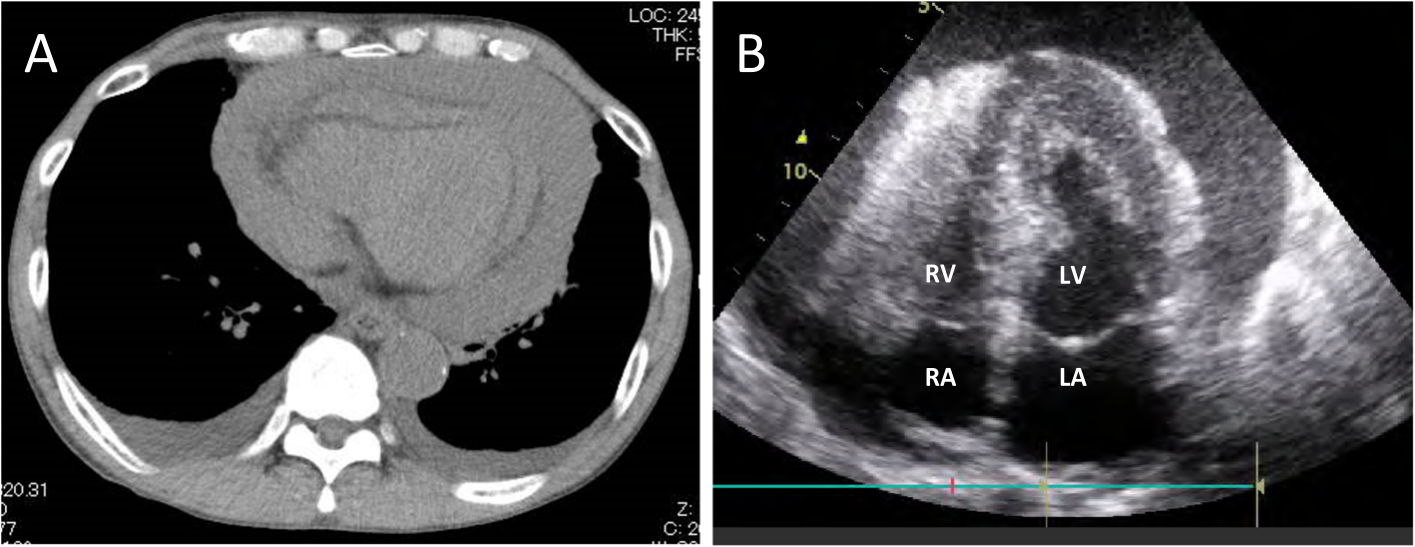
a Computed tomography findings. Extensive pericardial effusion and bilateral pleural effusion. b Echocardiography (apical four-chamber view) findings. An echo-free space around the heart revealed severe pericardial effusion. *LA* left atrium, *LV* left ventricle, *RA* right atrium, *RV* right ventricle
